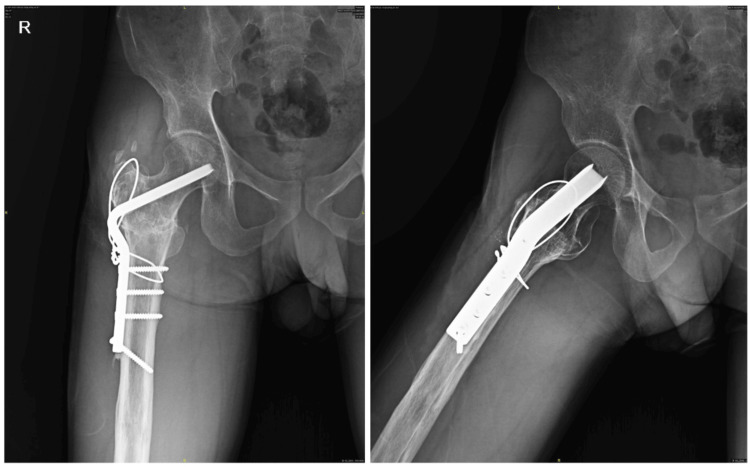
Four month follow-up X-ray of the right hip showing healed fracture after internal fixation with 120° angle blade plate
